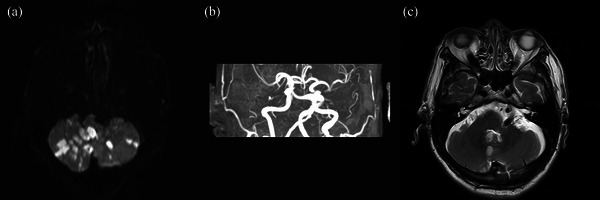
A male patient aged 70 years and complaining of “dizziness, nausea, unsteadiness, and tinnitus.” (a) DWI shows multiple infarcts in the bilateral cerebellar hemisphere. (b) MRA shows VBD. (c) Axial T2‐weighted images show that tortuous basilar artery compresses and pulls on the pons and VIII cranial nerve.
